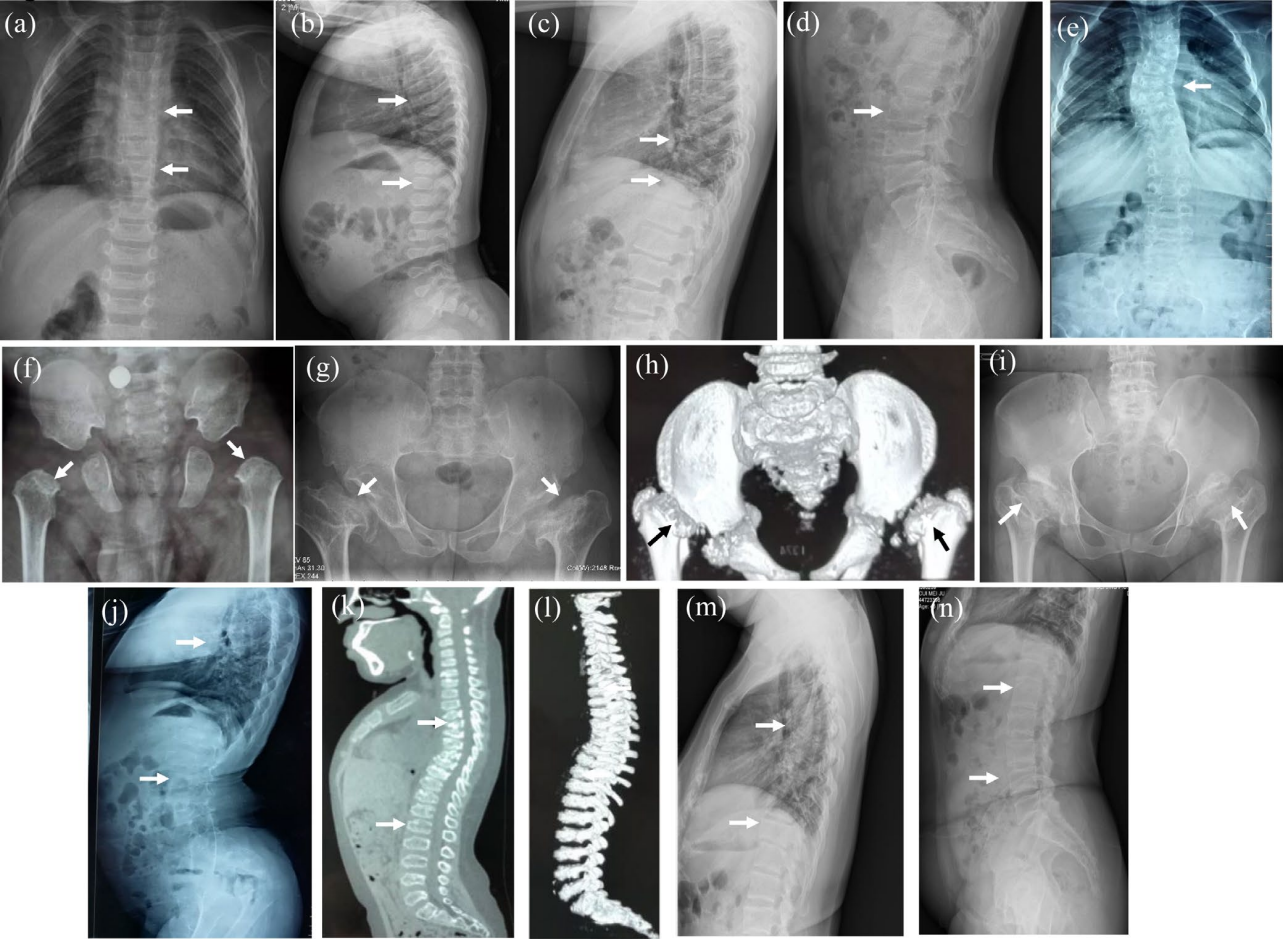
Imaging features of four spondyloepiphyseal dysplasia congenita patients with *COL2A1* variants. Proband 1. X‐ray films revealed flattened vertebral bodies, kyphosis, lumbar lordosis (a, b), flattened acetabular roof, compressed femoral heads, femoral heads epiphyses dysplasia and shortened femoral necks (f). Proband 1's mother. Radiographs revealed mild kyphosis, lumbar lordosis (c, d), flattened acetabular roof, and shortened femoral necks (g). Proband 2. Radiographs showed flattened vertebral bodies, kyphosis, lumbar lordosis and scoliosis (e, j–l), flattened acetabular roof, compressed femoral heads, femoral heads epiphyses dysplasia, and shortened femoral necks (h). Proband 2' father. X‐ray films revealed mild kyphosis, lumbar lordosis (m, n), flattened acetabular roof, and shortened femoral necks (i)
